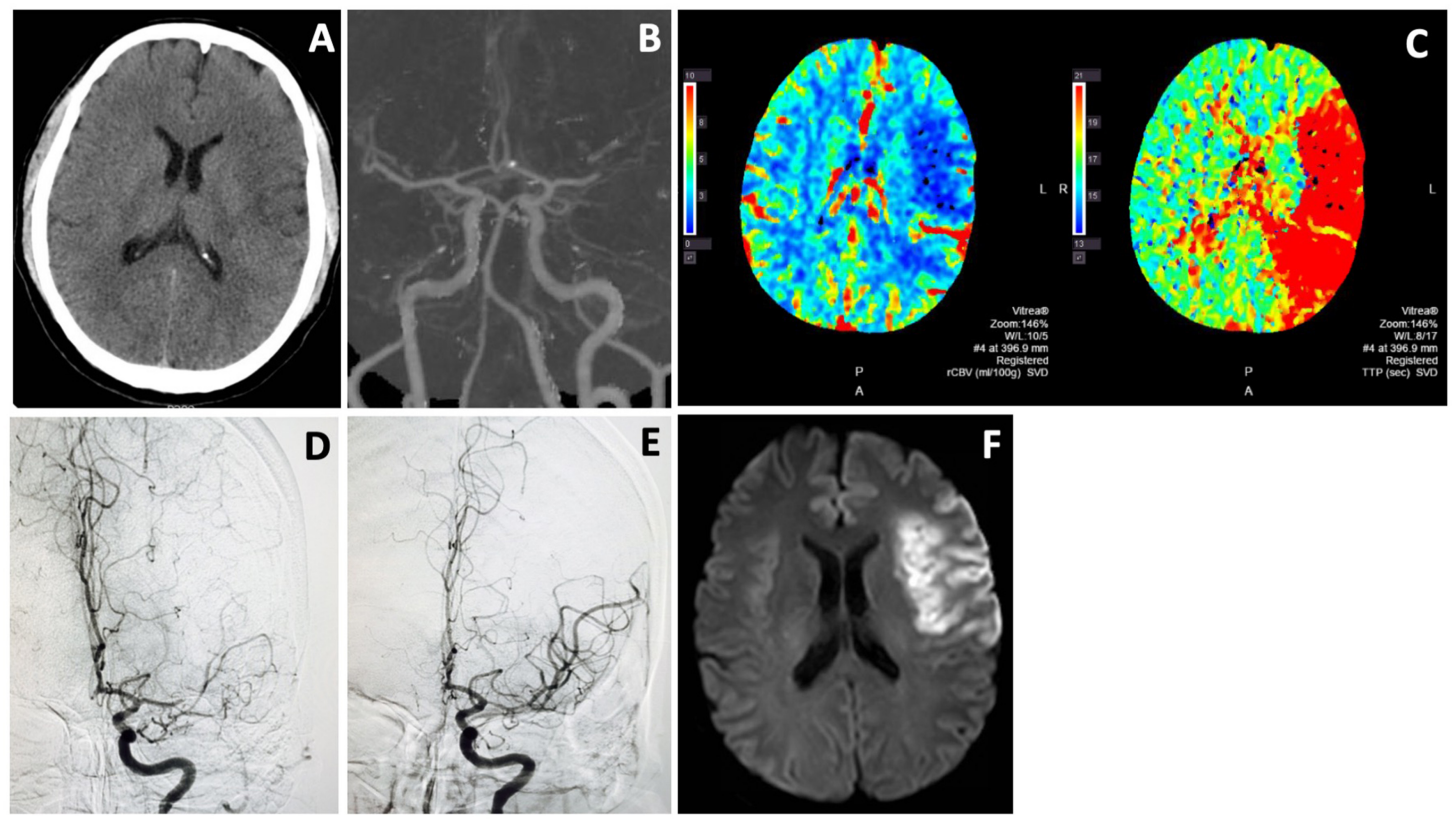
Endovascular thrombectomy for wake-up stroke. A 44 years old man woke up with right sided weakness and global aphasia. Last known well was 8 p.m. the night before. NIHSS score was 15. Non-contrast CT showed subtle left frontal hypodensity with ASPECTS of 8 (A). CTA showed L MCA M1 occlusion (B) and CTP revealed a large ischemic penumbra (C). He underwent thrombectomy with excellent MCA recanalization (D,E). Follow-up MRI (F) showed an infarct in the left frontal region that was similar in size to the hypodense area on non-contrast CT and infarct core on CTP. He recovered well with only mild expressive aphasia at 3 month.
